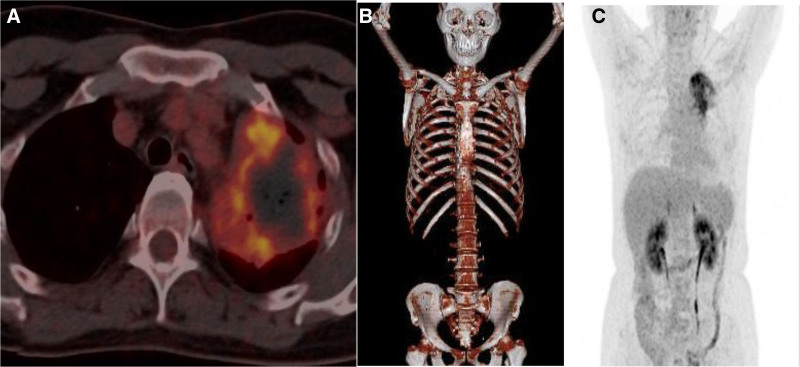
Above are the ^18^F-FDG-PET/CT images, where part (A) shows a high FDG uptake in the upper lobe of the left lung (standardized uptake value maximum of 6.3), and parts (B) and (C) are whole-body images. 18F-FDG PET/CT = ^18^F-fluorodeoxyglucose positron emission tomography/computed tomography, FDG.
